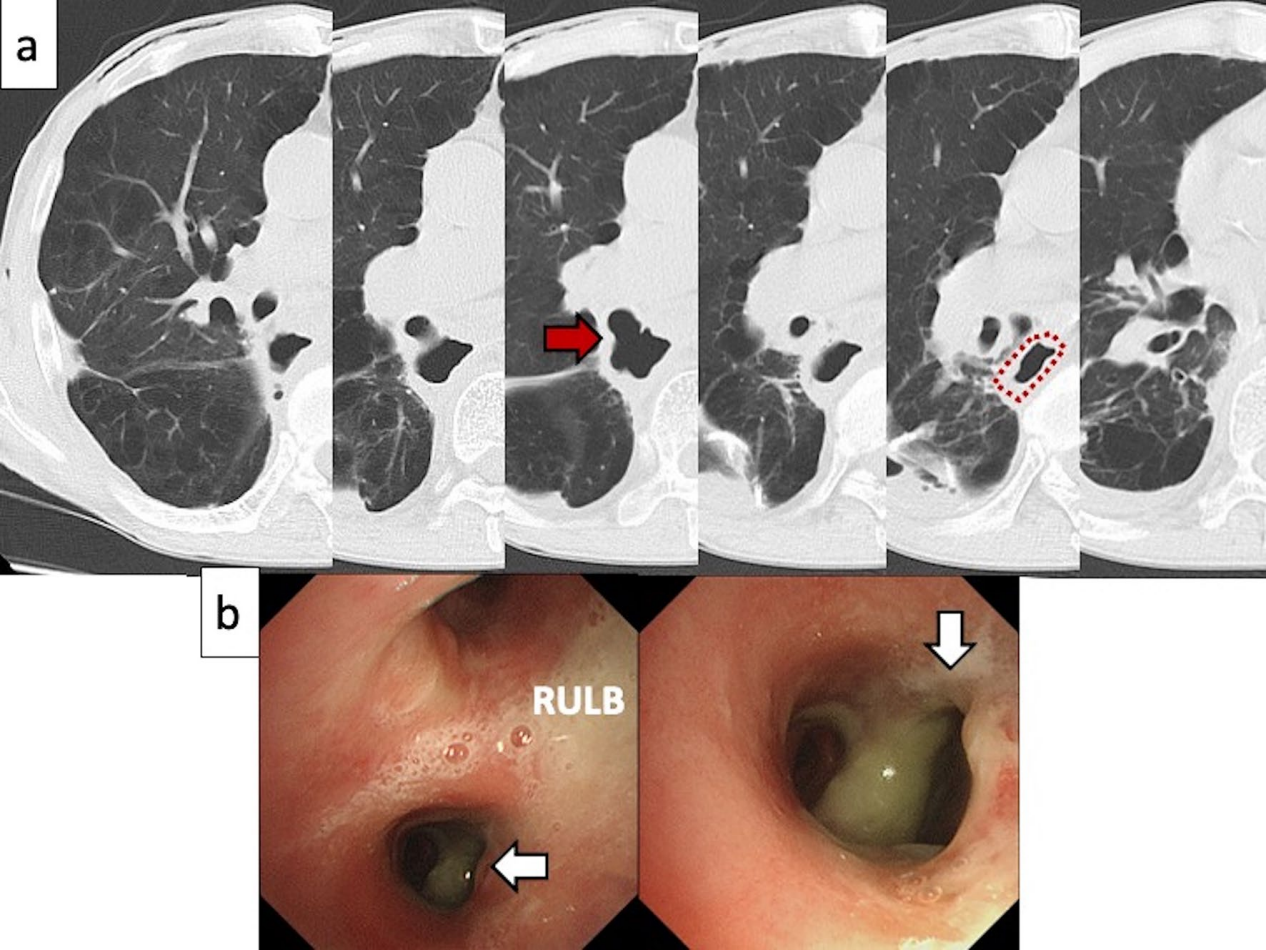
a Computed tomography on postoperative day 24 shows a fistula on the IBT (arrow) to the inside of the bulla in the segment 6 of the right lung (dotted line). b Bronchoscopic finding reveals a large perforation of the IBT (arrows). *RULB* right upper lobe bronchus
